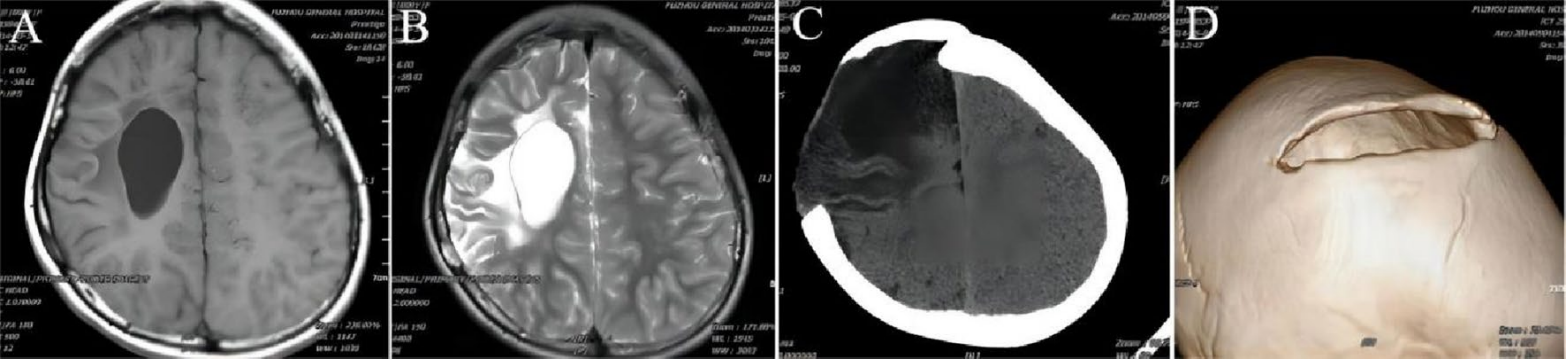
The imaging characteristics of Type IV. (A) MRI-T1WI showed lateral ventricle expansion. (B) MRI-T2WI showed atrophy and necrosis of the right parietal brain tissue. (C) CT showed a softening of the right parietal brain tissue, a local skull defect. (D) 3D-CT showed a “crater-like” change at the defect margin.
